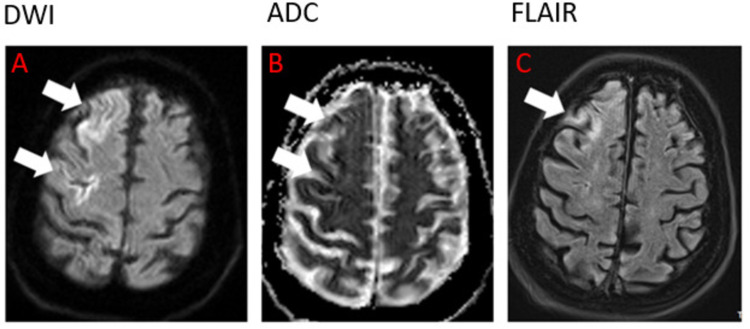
Brain MRI images (DWI, ADC, and FLAIR) showing an acute right frontal hemispheric stroke. DWI: diffusion-weighted imaging; ADC: apparent diffusion coefficient; FLAIR: fluid-attenuated inversion recovery
